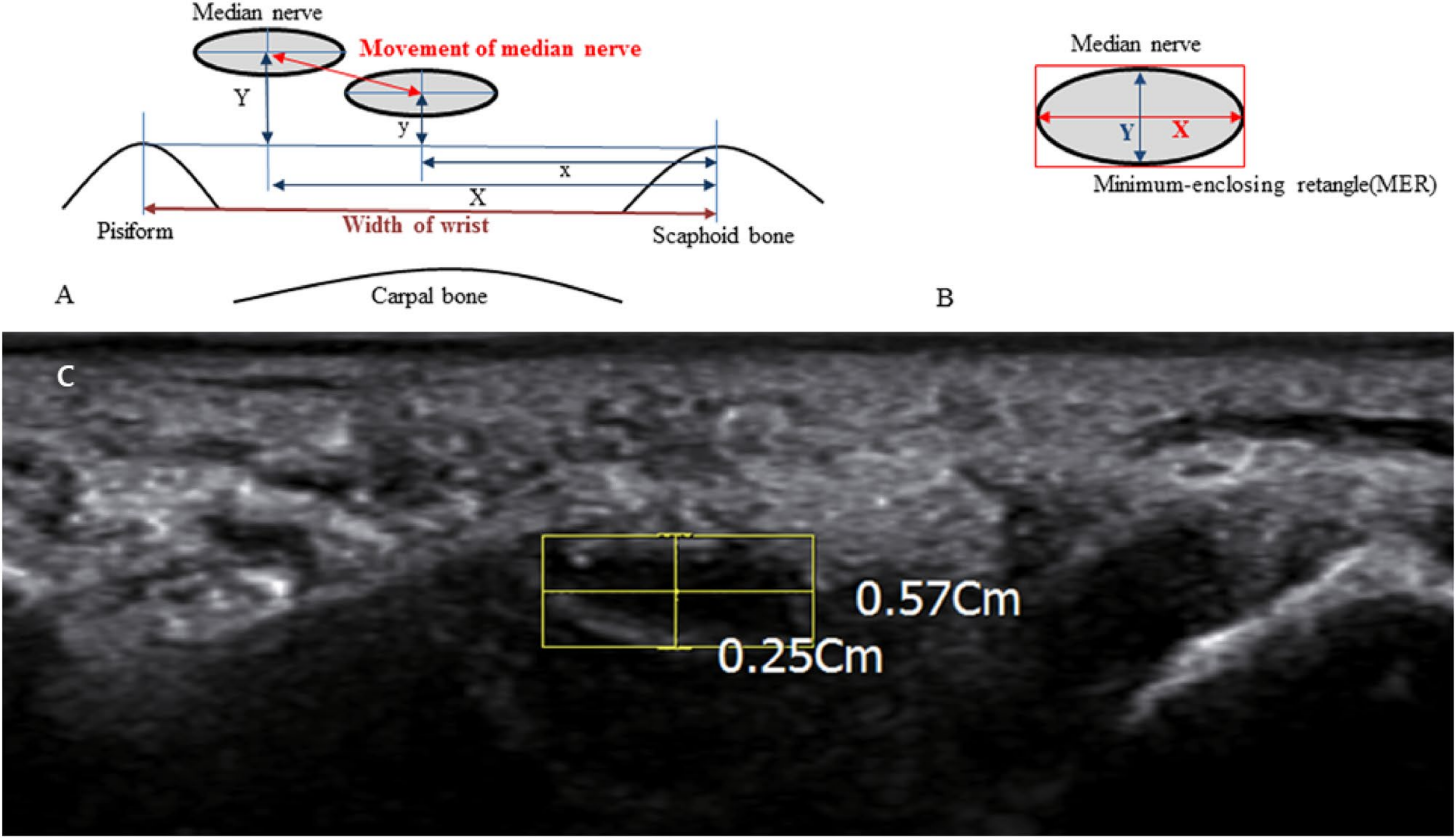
(A) Ultrasound measurement of transverse median nerve displacement in response to wrist and finger movement. To calculate the maximal change value of the median nerve displacement in response to finger and wrist movements, the maximal and minimal displacement of the median nerve from the scaphoid tubercle were chosen during six motions (the maximal displacement of the median nerve from the scaphoid tubercle = $$\sqrt {{\text{X}}^{2} + {\text{Y}}^{2} } ;$$ the minimal amplitude of the median nerve from the scaphoid tubercle = $$\sqrt {{\text{x}}^{2} + {\text{y}}^{2} }$$). The maximal change in the median nerve displacement was defined as: $$\sqrt {\left( {X - x} \right)^{2} + \left( {{\text{Y}} - {\text{y}}} \right)^{2} }$$. (B,C) The measurement method of the aspect ratio of the minimum-enclosing rectangle (MER). The aspect ratio of the MER was defined as $$\frac{X \;(Major \;axis\; of\;MER)}{{Y\;\left( {Minor \;axis \;of\; MER} \right)}}$$.
